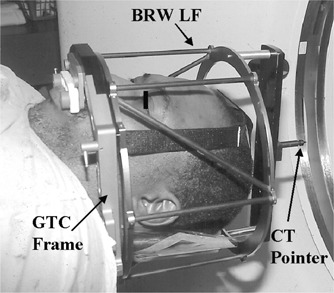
CT scanning of a patient. The GTC relocatable frame contains fiducial marks for both SRT and IMRT.
